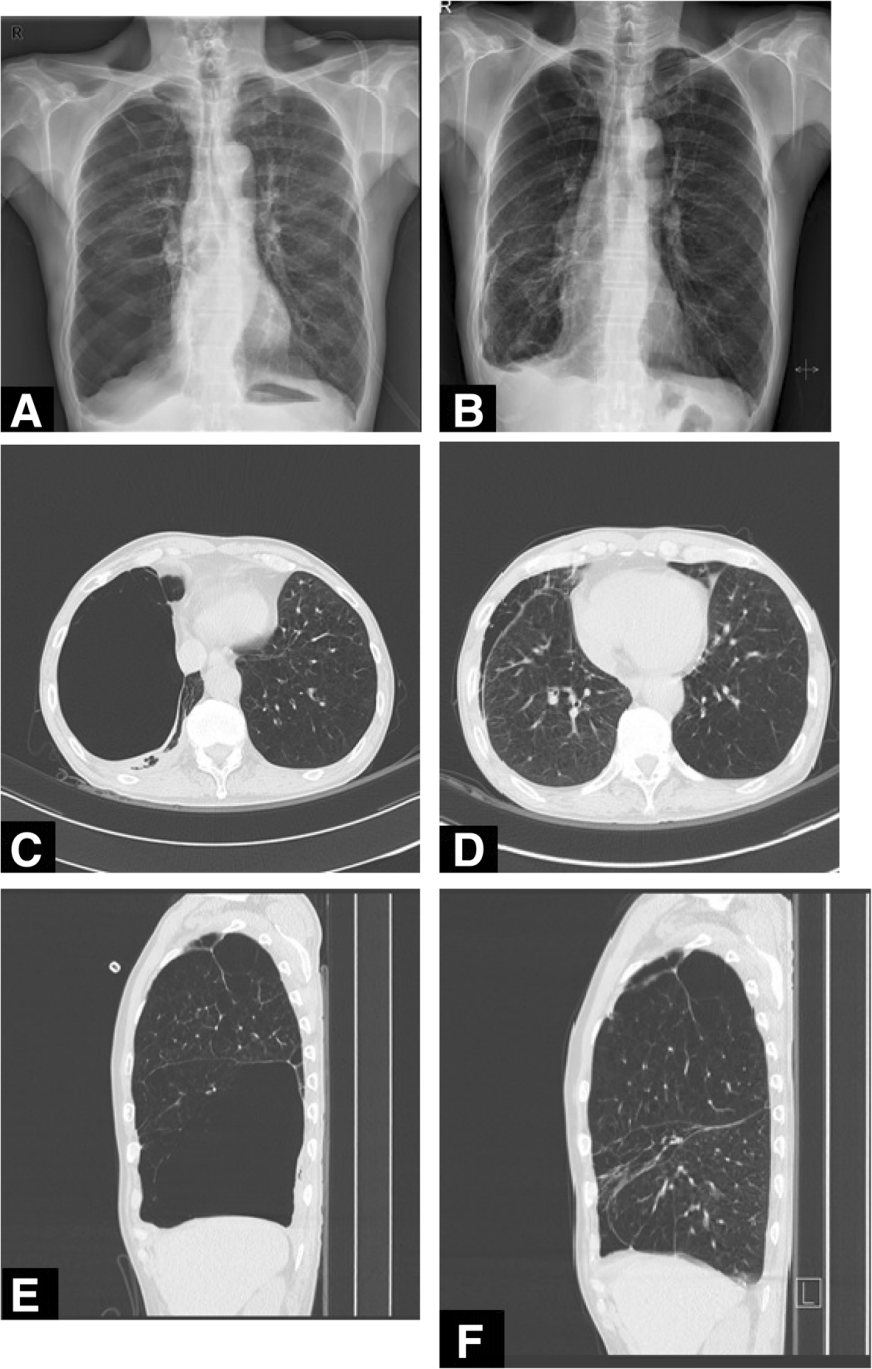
a, c and e Chest X-ray taken before the procedure (November, 2017) and computed tomography (CT) scan taken in May, 2017 (transverse and sagittal views, respectively) indicated severe emphysema and a huge bullae in the right middle lobe compressing the right lower lobe parenchyma. b, d and f A Chest X-ray taken 1 week after the procedure (November, 2017) and a CT scan taken 2 months after the procedure (January, 2018, transverse and sagittal views, respectively) showed that the huge bullae in the right middle lobe had disappeared. This caused the volume of the right middle lobe to decrease but that of the compressed right lower lobe to re-expand
